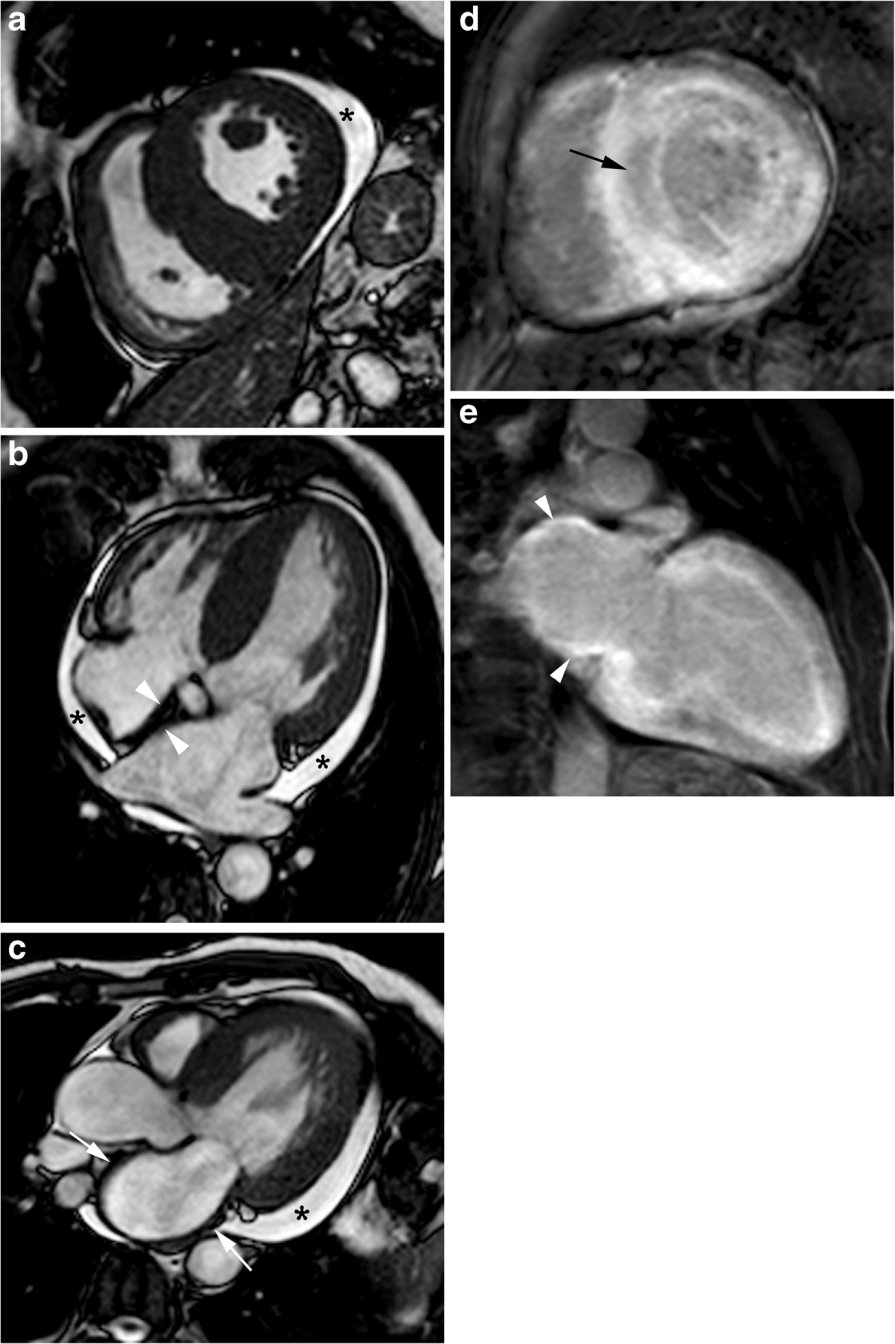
A 65-year-old man with cardiac amyloidosis. a Short-axis, (b) four-chamber and (c) three-chamber cine SSFP MR images at end-diastole show diffuse asymmetric myocardial thickening, of both, LV and RV, thickening of left atrial wall (*arrows*) and interatrial septum (*arrowheads*) and pericardial effusion (*asterisks*). d Short-axis and (e) two-chamber LGE images showing transmural atrial wall enhancement (*arrowheads*) and diffuse myocardial enhancement sparing the mid-wall of ventricular septum (*arrow*)
